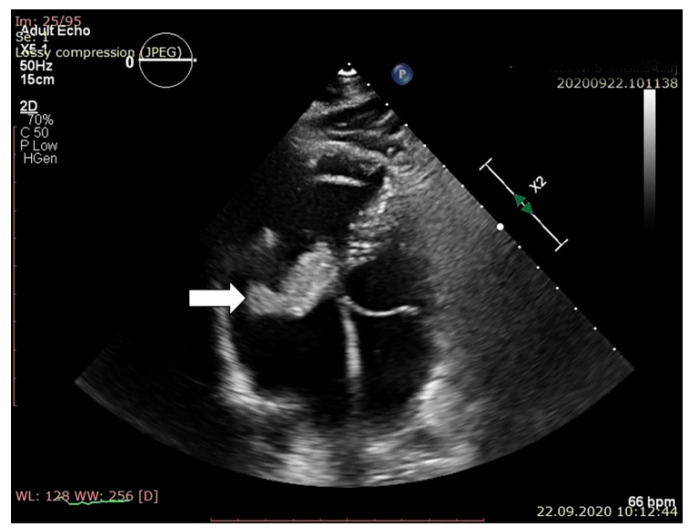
Apical 4 chamber view. Systolic prolapsed in the right atrium of the septal leaflet and vegetation.
